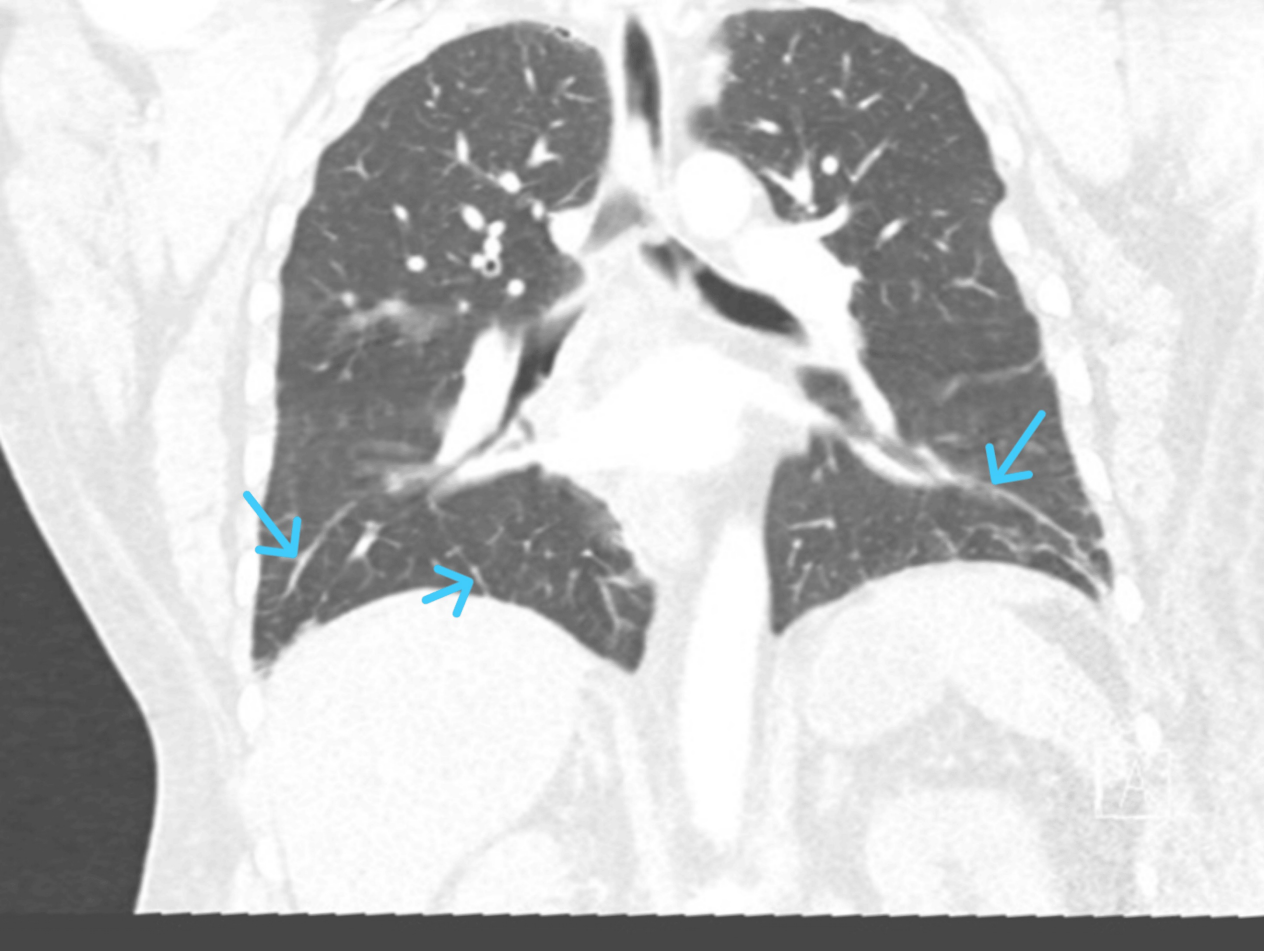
CT pulmonary angiogram on admission showing bi-basal interlobular septal thickening consistent with pulmonary edema (blue arrows).
